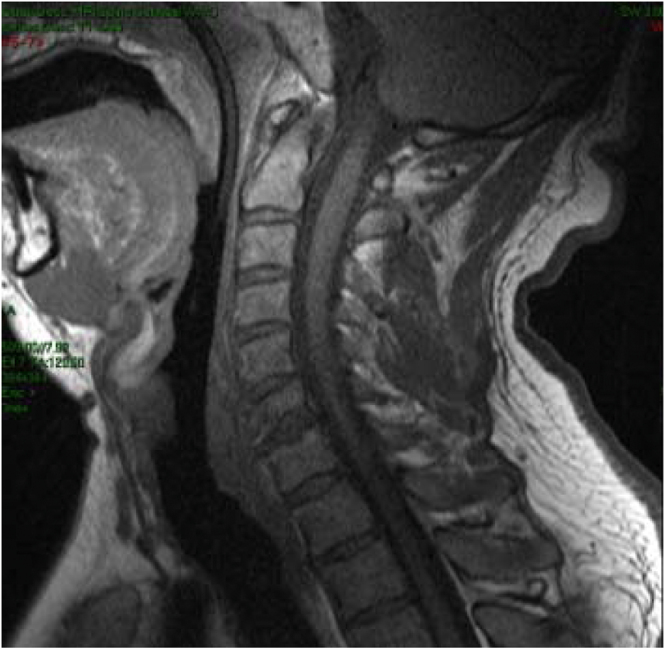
MRI cervical spine with and without contrast shows no evidence of cord compression and degenerative changes.
